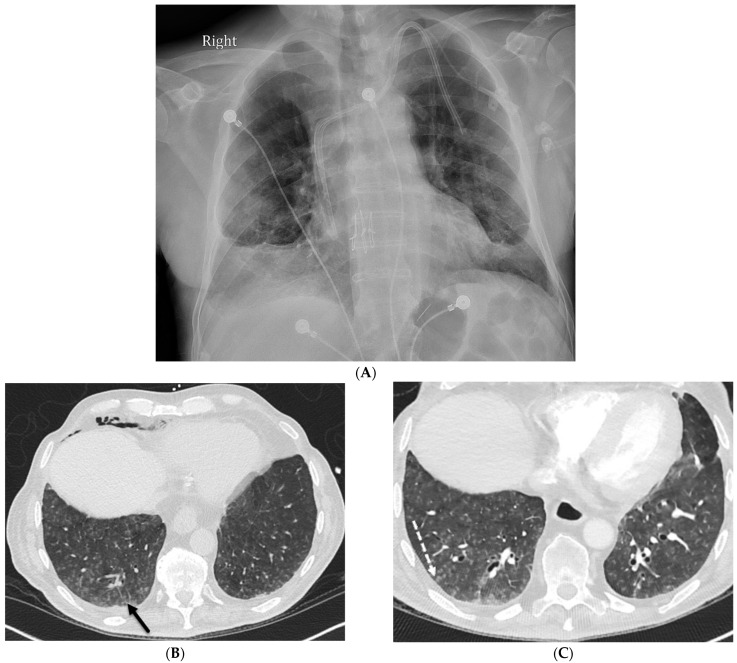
A 68-year-old male with a history of idiopathic pulmonary fibrosis underwent bilateral lung transplantation and now presents with shortness of breath and hypoxia five months later. Bronchoscopy revealed adenovirus infection. The frontal chest on the right in (A) demonstrates interstitial prominence. Axial chest CT images (B,C) reveal tree-in-bud opacities (dashed white arrow) in both lower lobes and smooth interlobular septal thickening (solid black arrow).
